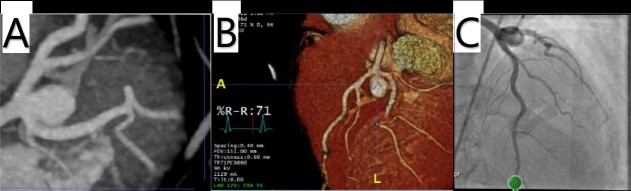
(A, B) Cardiac CTA showing a saccular aneurysm measuring 12.5 mm in length from the LMCA bifurcation to the apex of the aneurysm, and 9.04 × 10.5 mm in cross-sectional area. (C) LHC showing a distal LMCA aneurysm between the LAD and left circumflex.
